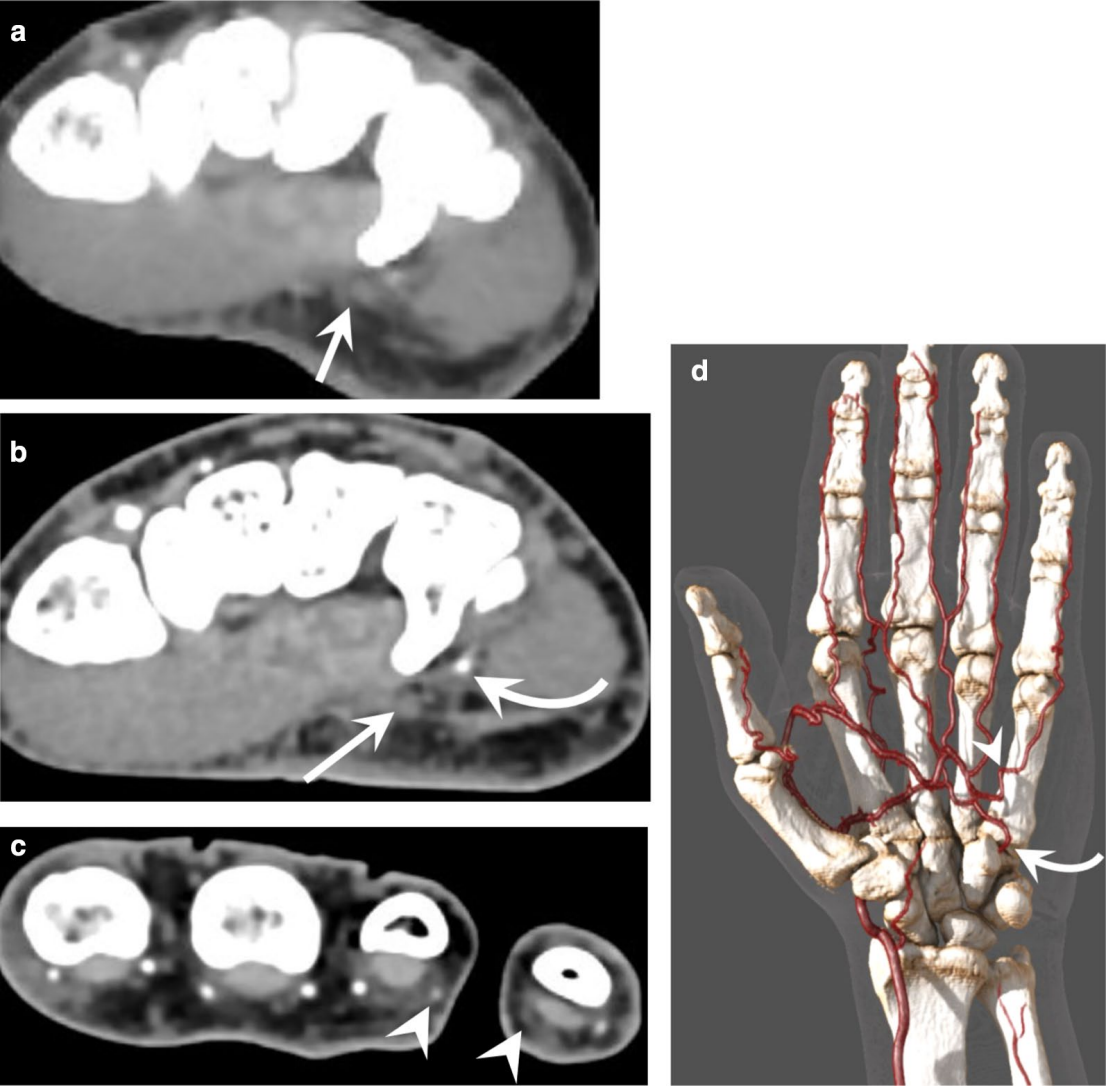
Comparison of the image quality between a complete upper extremity run-off and a CTA of the hand in a 52-year-old patient with HHS, presenting with ischaemia of the fourth and fifth fingers. a A complete upper extremity run-off was first obtained showing a probable occlusion of the UA. b, c CTA of the hand showed a 6-cm-long occlusion of the UA, the patency of the deep branch of the UA (curved arrow), which is fed by the DPA, the occlusion of the ulnopalmar artery of the fifth finger and the distal occlusion of the radiopalmar artery of the fourth finger (arrowheads). d GI providing an overview of the lesions and of the arterial configuration of the hand showing the deep branch of the UA (curved arrow) and the occlusion of the ulnopalmar artery of the fifth finger (arrowhead)
